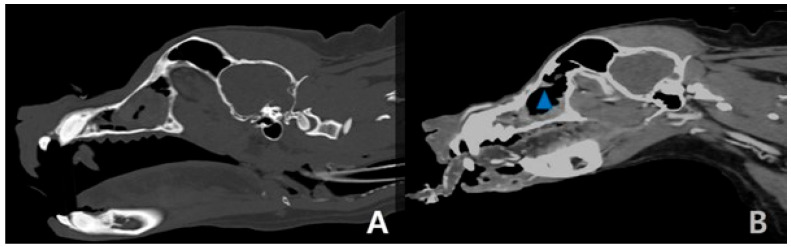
(A,B) Sagittal view of the CT scan of the skull. The size of the nasal tumor decreased significantly (blue triangle).
